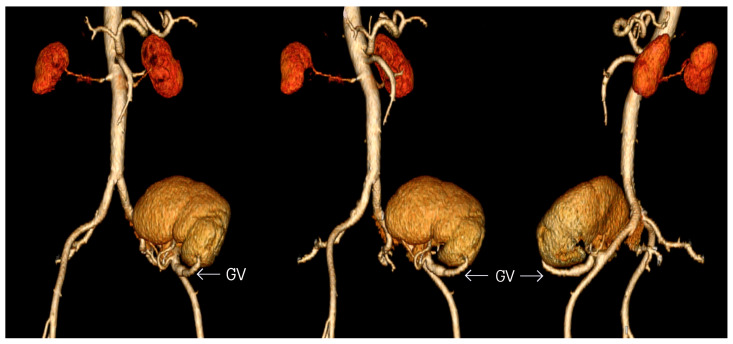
CT angiography with aortoiliac reconstruction after kidney transplantation. GV, gonadal vein.
